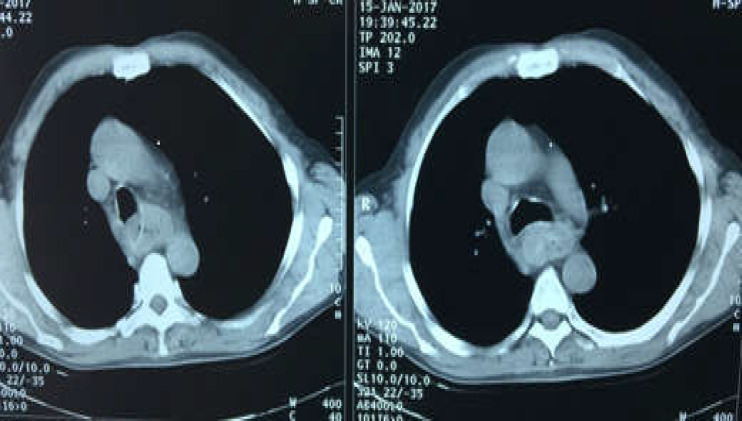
Computed tomography revealed hypodense foci in the mediastinum without definitive margins plus esophageal prominent mucosal pattern
